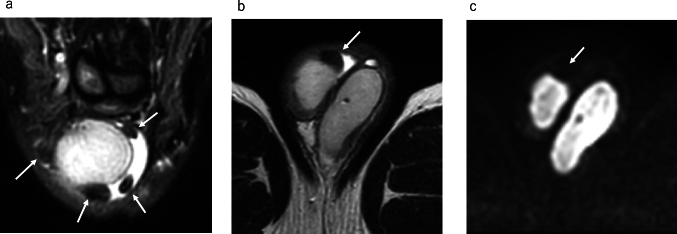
Fibrous pseudotumor in a 53-year-old male with right paratesticular masses accompanied with mild hydrocele. a Coronal fat-suppressed T2-weighted image demonstrates hypointense masses (white arrows). b Axial T2-weighted image shows a hypointense mass attaching to the tunica vaginalis (white arrow). c Axial diffusion weighted MR image shows a hypointense mass (white arrow), and it is difficult to identify the location of mass without referring to other sequencing images
